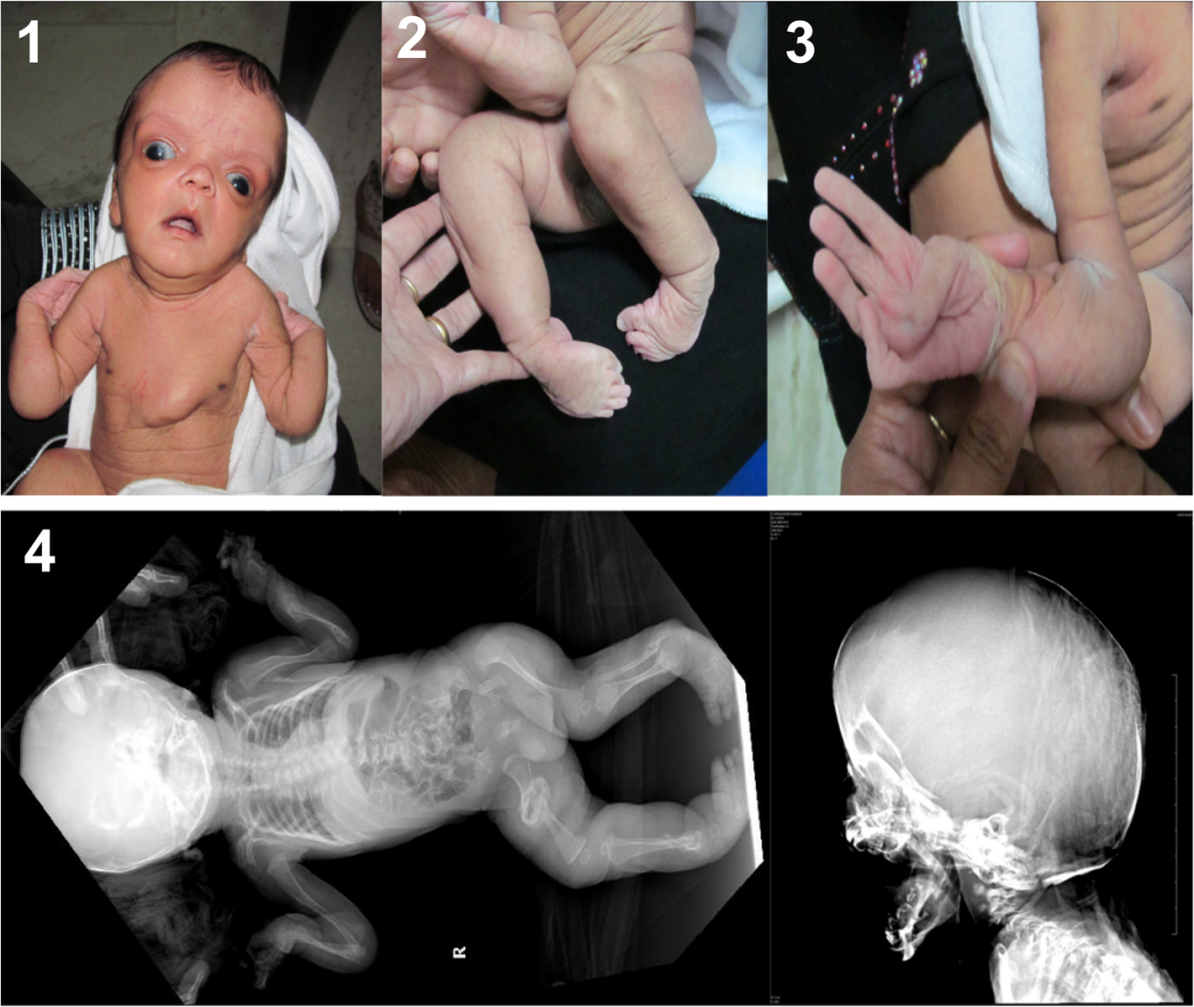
Patient 1 with severe dysmorphic features. There is bulging of the thoracic cage, hypertolerism, downslanting palpebral fissures, lagophtalmos of the lower eye lids, blue sclerae, a pug nose, low-set and dysplastic ears, corneal clouding [1], generalized cutis laxa [1–3], long fingers with campylodactyly and adducted thumbs, broad tips of fingers and toes and bilateral club feet [2, 3]. Perinatal radiography shows osteopenia, multiple fractures, large joint contractures and Wormian bones in the occipital region [4]
